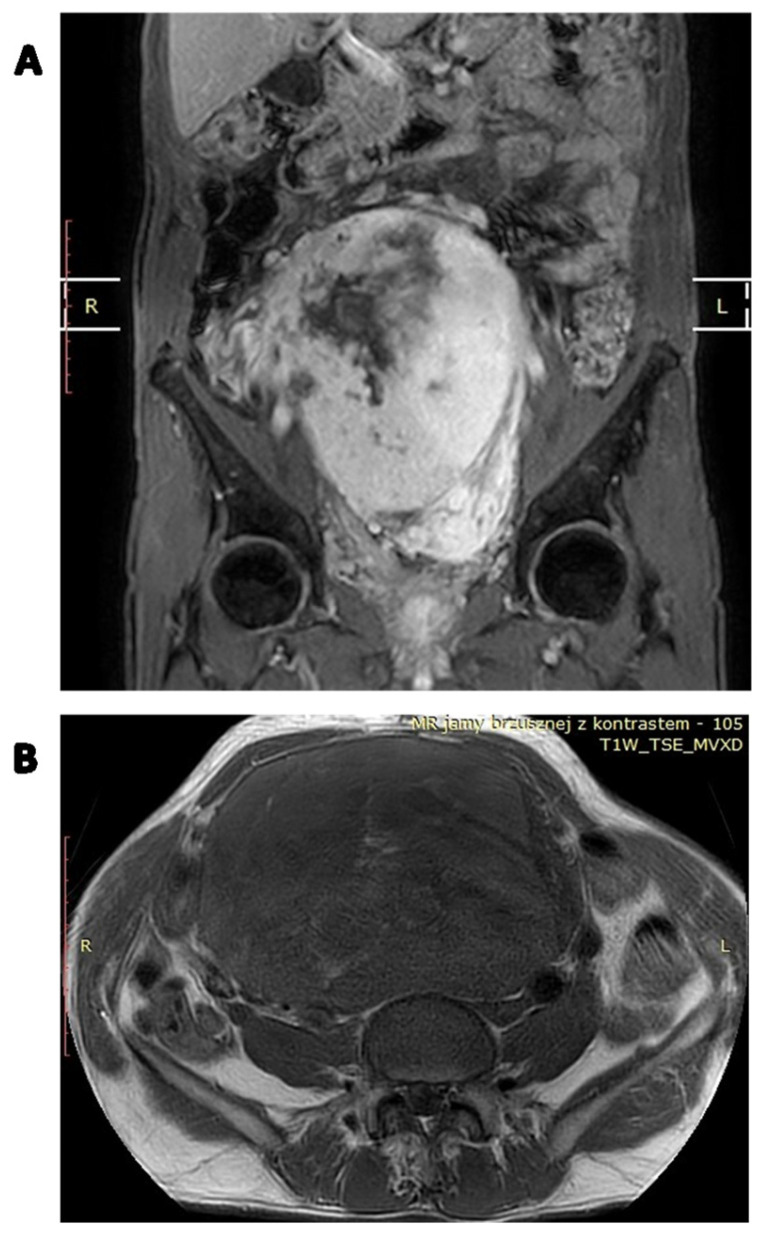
Contrast-enhanced MRI image of a locally advanced abdominal SFT: (A) coronal image; (B) axial image.
